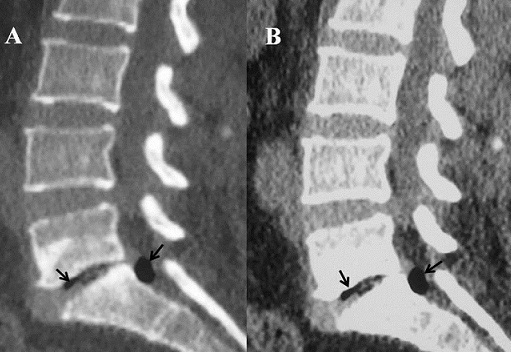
CT sagittal reconstruction (A, B) revealed vacuum phenomenon in intervertebral disc (arrow) with moderate disc protrusion at this level
